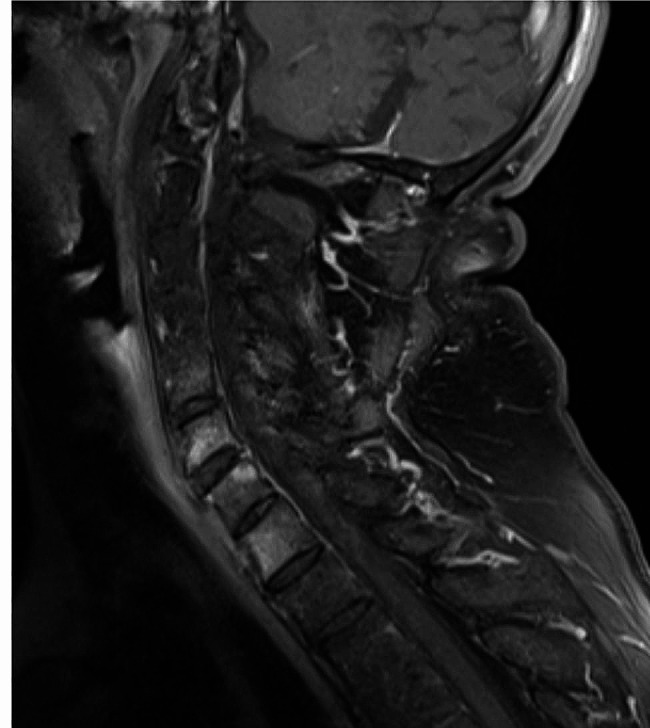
Bone marrow edema in the vertebral bodies. Sagittal MRI (T1- weighted with contrast agent), showing bone marrow edema in the vertebral bodies, mainly located in the area of the vertebral corner (arrow) of a patient with ankylosing spondylitis
